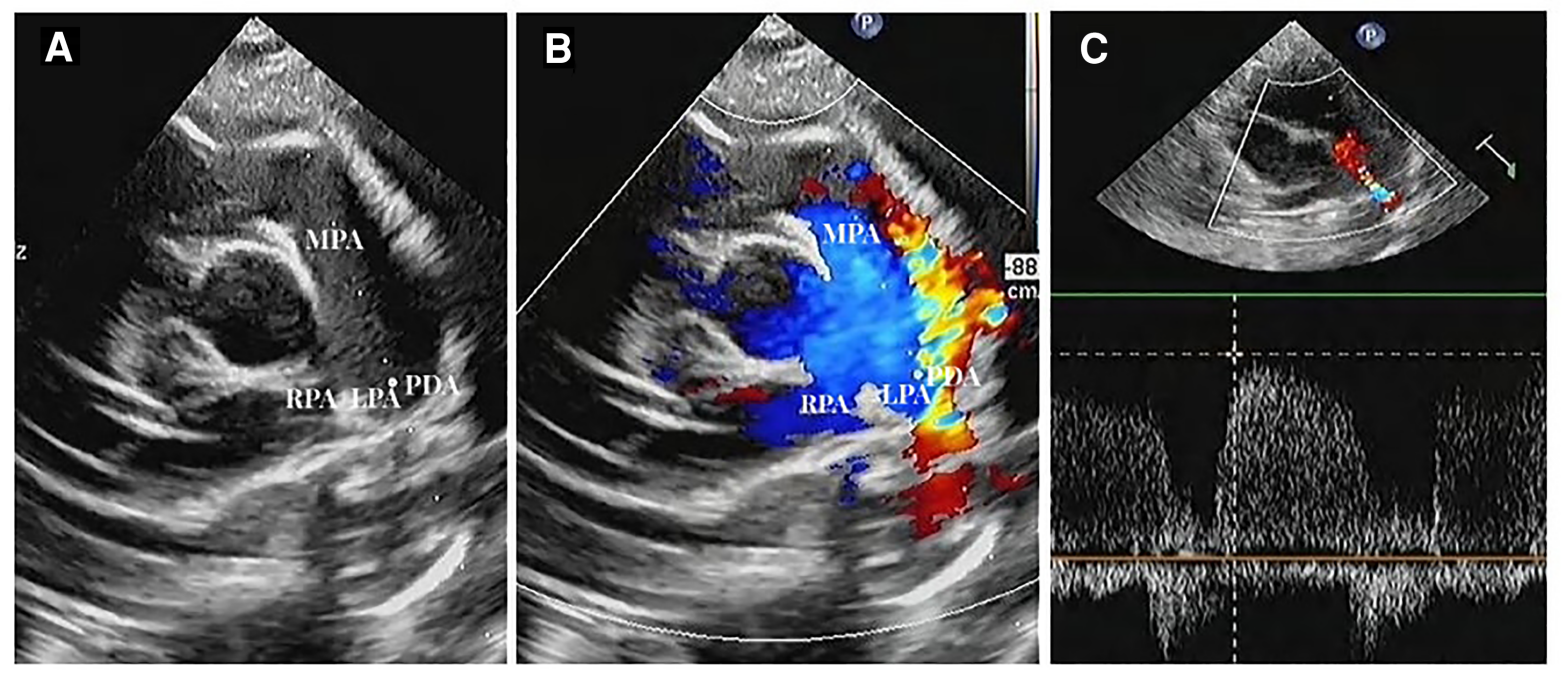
Ductus arteriosus in short axis view (A). Two-dimensional sonogram image; (B). Color Doppler sonogram image; (C). PW Doppler across the PDA flow (MPA, main pulmonary artery; LPA, left pulmonary artery; RPA, right pulmonary artery; PDA, unclosed ductus arteriosus).
